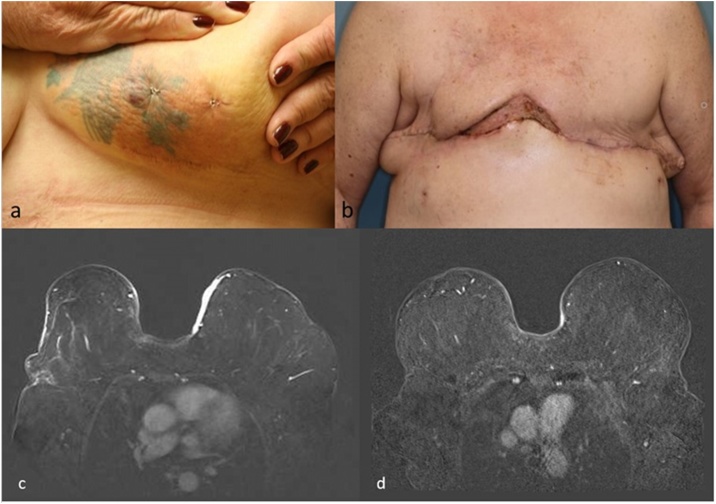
(a) AS of the left, medial breast, prior to chemotherapy. (b) Post bilateral, in-continuity mastectomies with resection of the suprasternal tissue. (c) MRI of bilateral breast AS at first presentation. (d) MRI after neoadjuvant chemotherapy.
